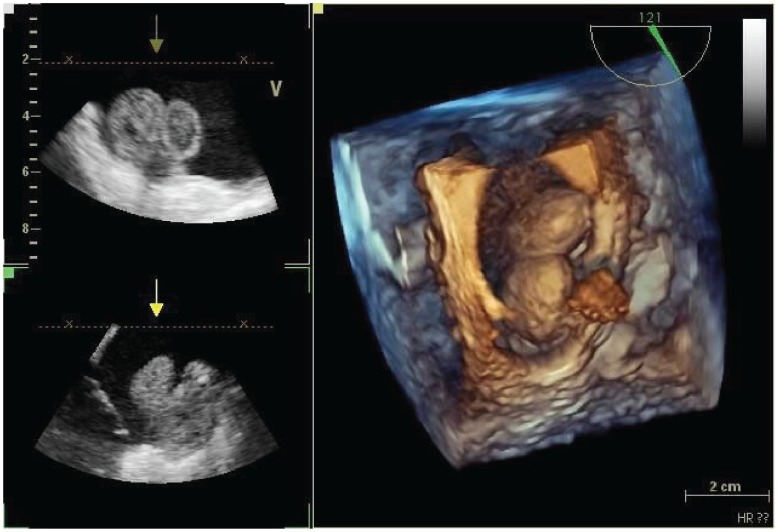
Tridimensional transesophageal echocardiogram of the mass in the right atrium.
